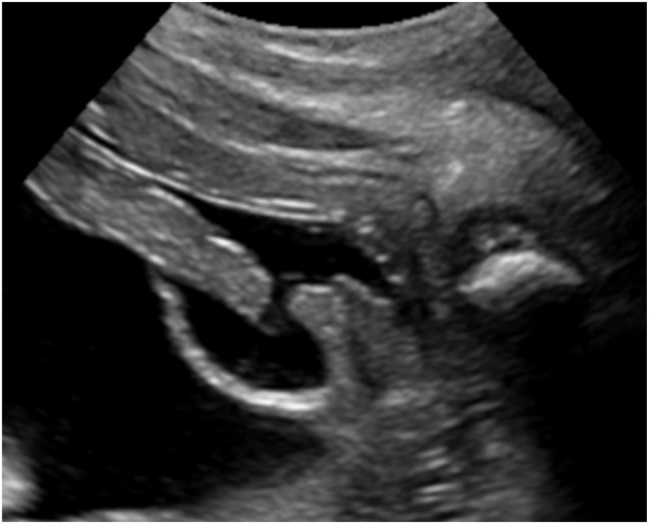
4th postpartal hour: ultrasound displaying a collapsed bladder with dorsal wall-defect, adherend web (peritoneum) and abdominal ascites.
